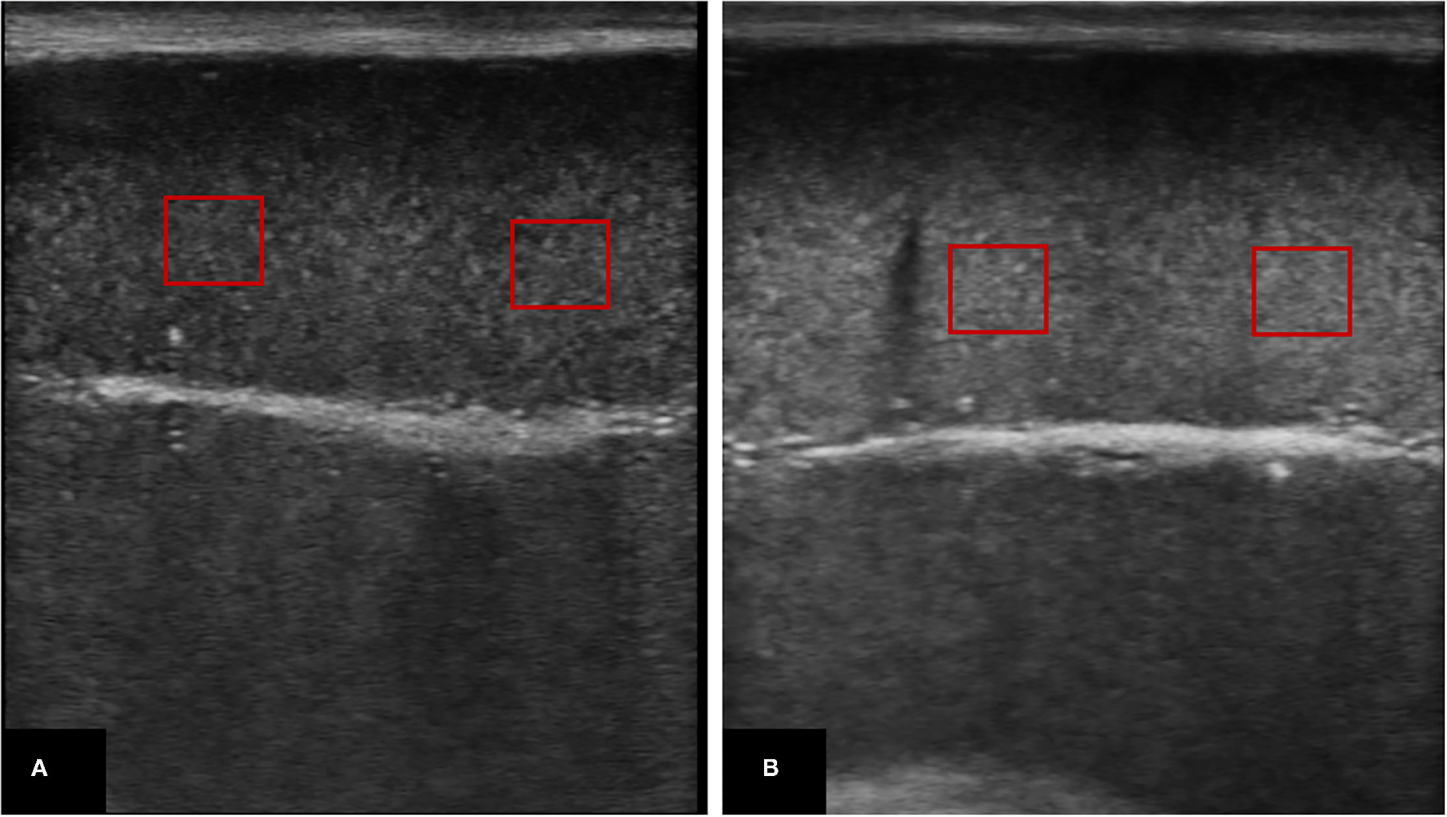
Ultrasonograms of the testicular parenchyma in which echotextures were assessed by placing at least 3 squares (1 cm × 1 cm) and underwent computed digital analysis (Adobe Photoshop 64 CC software); (A) represents testicular parenchyma with low echotexture, whereas (B) represents higher echotexture.
